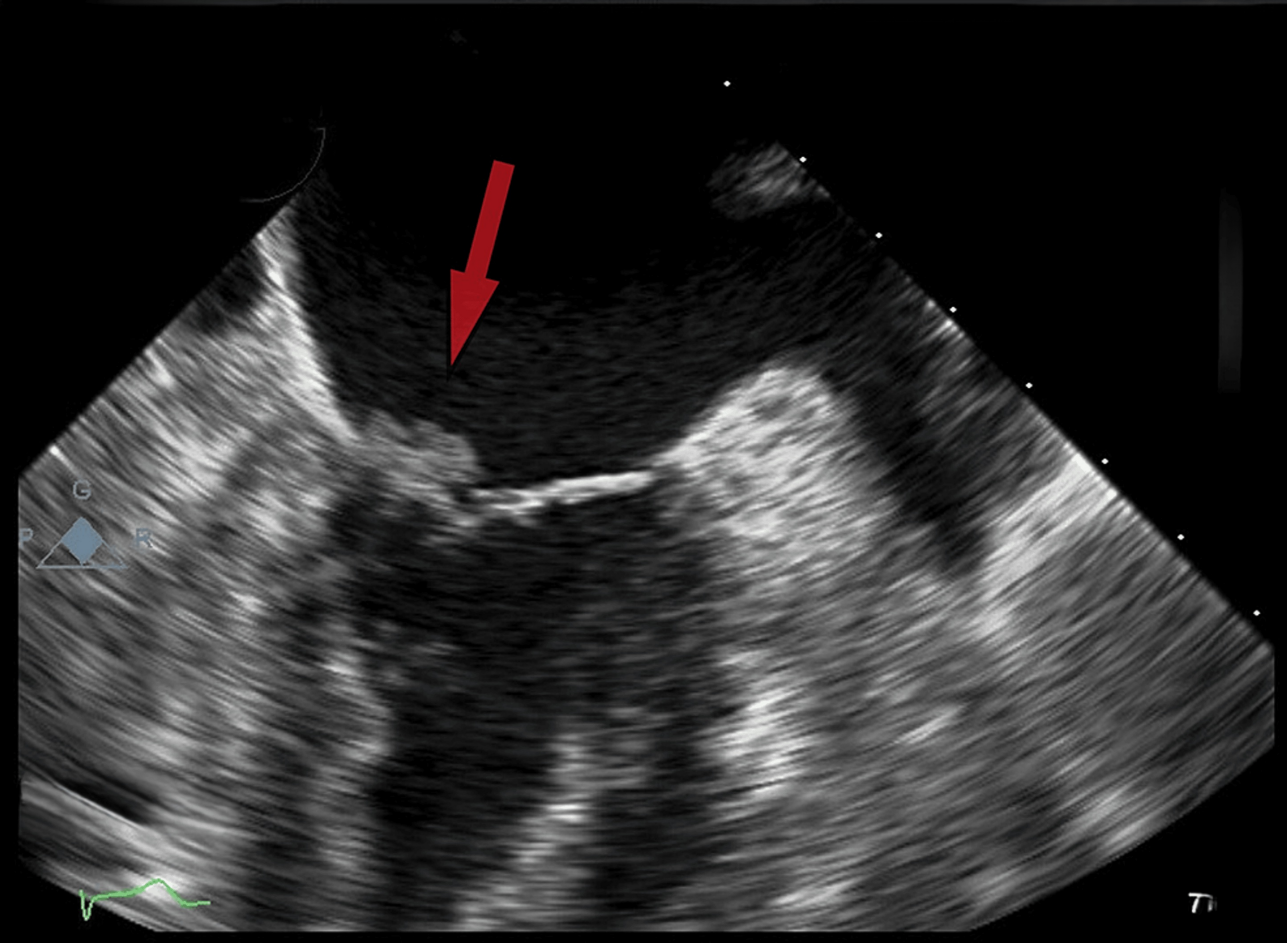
Transthoracic echocardiogram showing vegetations on the anterior mitral valve leaflet (arrow).
